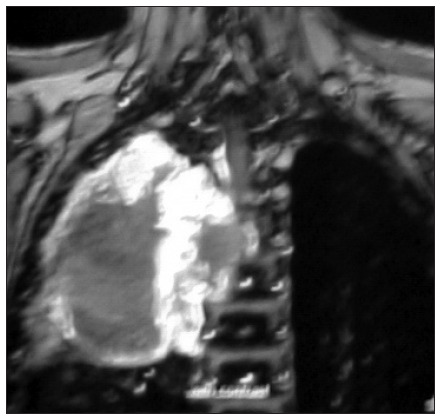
Chest T1-magnetic resonance imaging with gadolinium: a large mass with heterogeneous enhancement of solid components and necrotic non-enhancing component is seen in the right upper lobe, with destruction of vertebrae and invasion into the spinal canal, thereby compressing the spinal cord.
